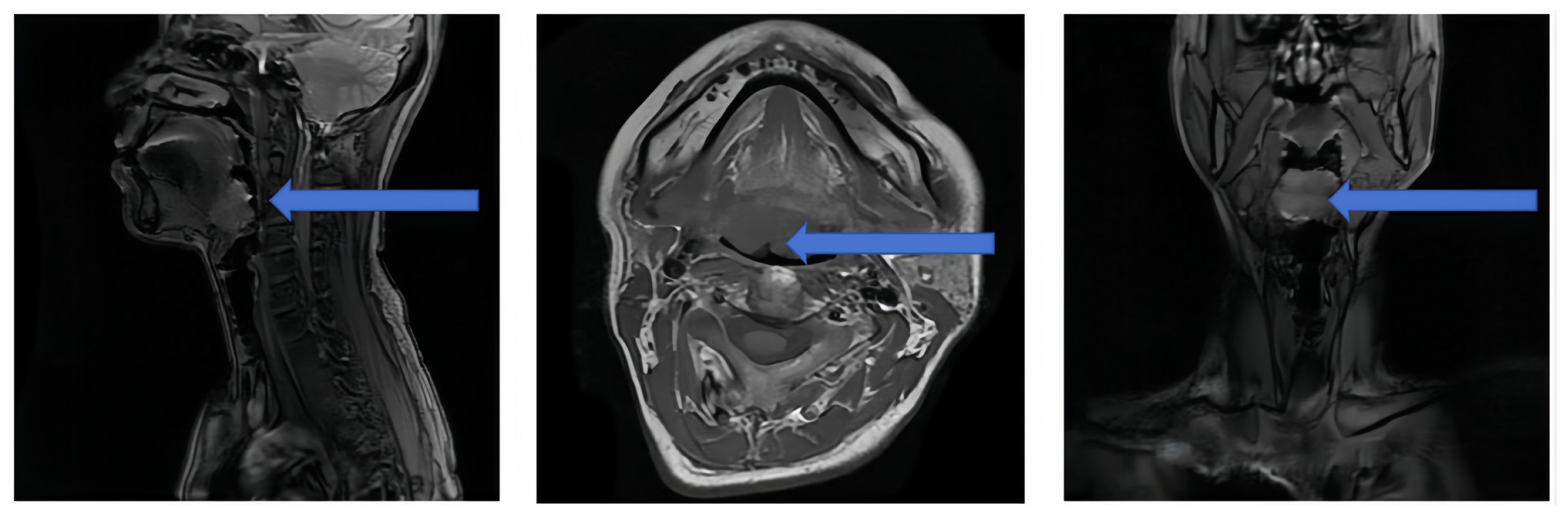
Preoperative laryngeal magnetic resonance imaging of the patient. The image revealed a soft tissue mass shadow on the right side of the posterior part of the tongue root with slightly high signal intensity on the T2WI fat-compression image (arrow), along with a linear isointense shadow within the lesion. The lesion measured ~3.5×2.3 cm, with compression of the epiglottic vallecula. The enhancement scan showed significant homogeneous lesion enhancement and no obviously enlarged lymph nodes were observed bilaterally in the neck.
